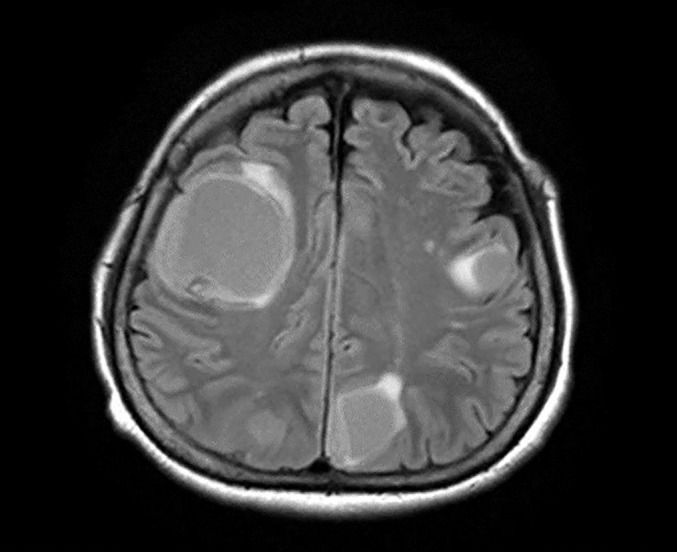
T2-weighted cerebral MRI revealed high-signal-intensity lesions with cysts in the bilateral cerebral hemispheres.
